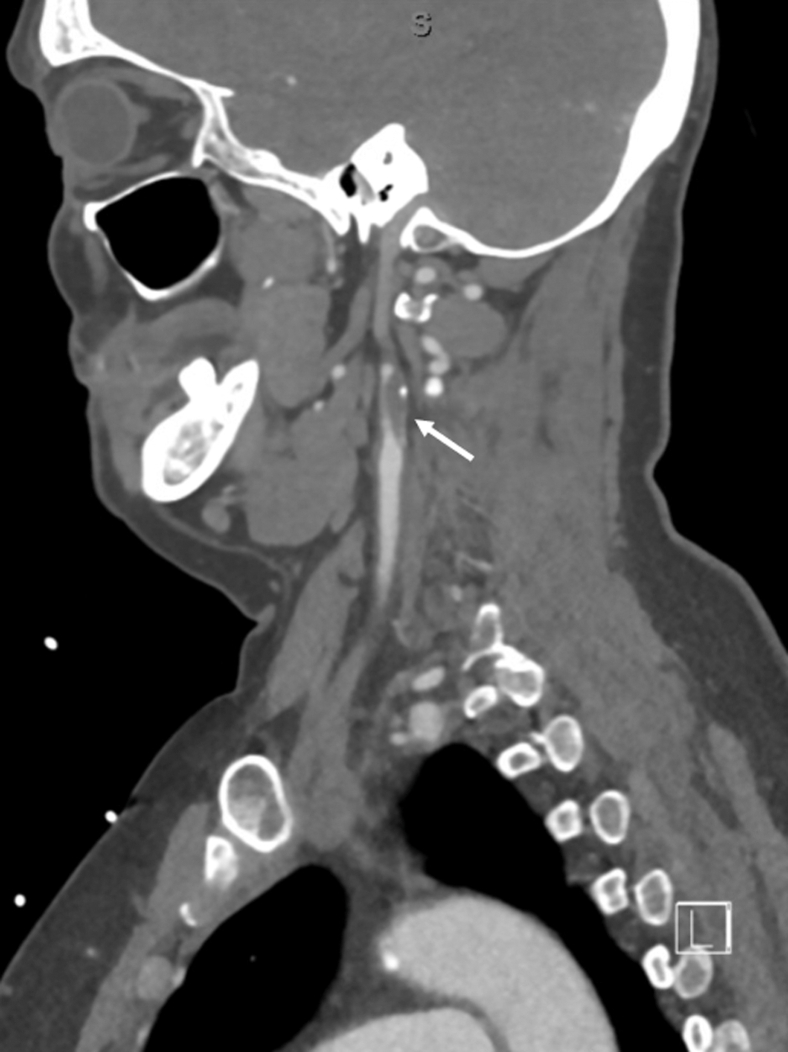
Sagittal view of a CT angiography of the head and neck. The arrow points to the left internal carotid artery in an area with 95% stenosis. The patient is noted to have both a hard and soft plaque in the area of stenosis. CT, computed tomography.
